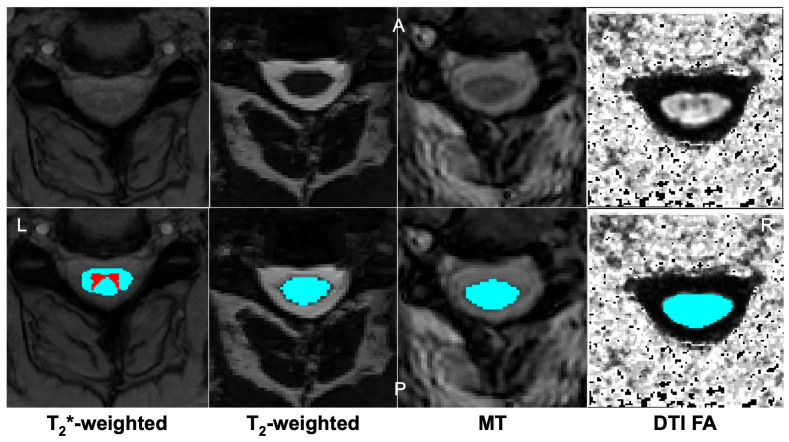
MRI scan visualization and automated segmentation using the Spinal Cord Toolbox. Images were captured at the C3 level from a representative control patient. The top thumbnails show the image without segmentation, and the bottom images include segmentation. (Left to Right) T_2_*-weighted grey matter (red) and white matter (blue) scan, T_2_-weighted, magnetization transfer scan, and fractional anisotropy [69]. MT = magnetization transfer, DTI = diffusion tensor imaging, L = left, R = right, A = anterior, P = posterior.
